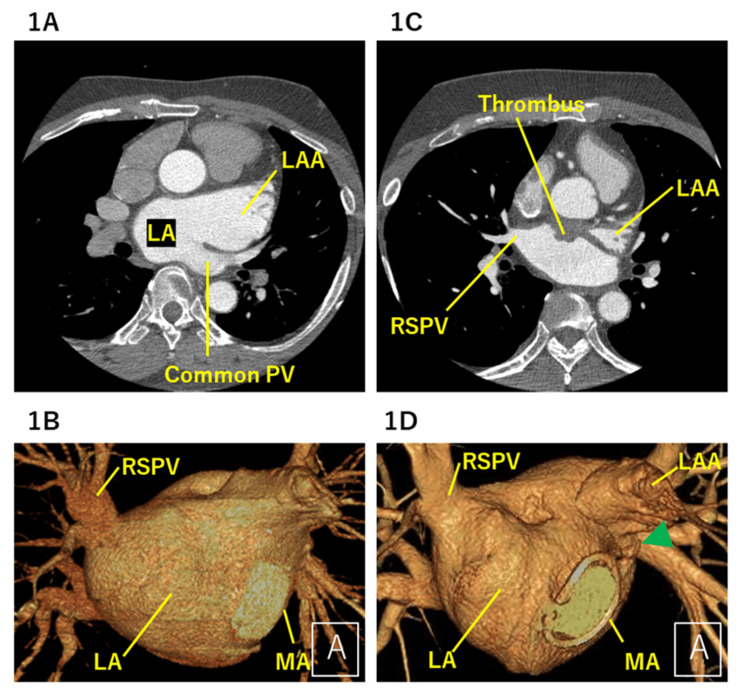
Contrast-enhanced CT before and after surgical LAAC (A) Axial contrast-enhanced CT image obtained before cardiac surgery demonstrating a markedly enlarged LA, LAA, and a left common pulmonary vein. (B) Three-dimensional CT reconstruction preoperatively showing the anatomical relationships among the LA, LAA, mitral annulus, and pulmonary veins. (C) Axial contrast-enhanced CT image obtained approximately one year after surgical LAAC, demonstrating contrast opacification within the LAA and a mural filling defect along the anterior left atrial wall adjacent to the closure site. (D) Three-dimensional CT reconstruction after LAAC demonstrates continuity between the closure site and the remnant LAA, as well as the spatial relationship to adjacent left atrial structures. CT: computed tomography, LA: left atrium, LAA: left atrial appendage, LAAC: left atrial appendage closure, PV: pulmonary vein, RSPV: right superior pulmonary vein, MA: mitral annulus
